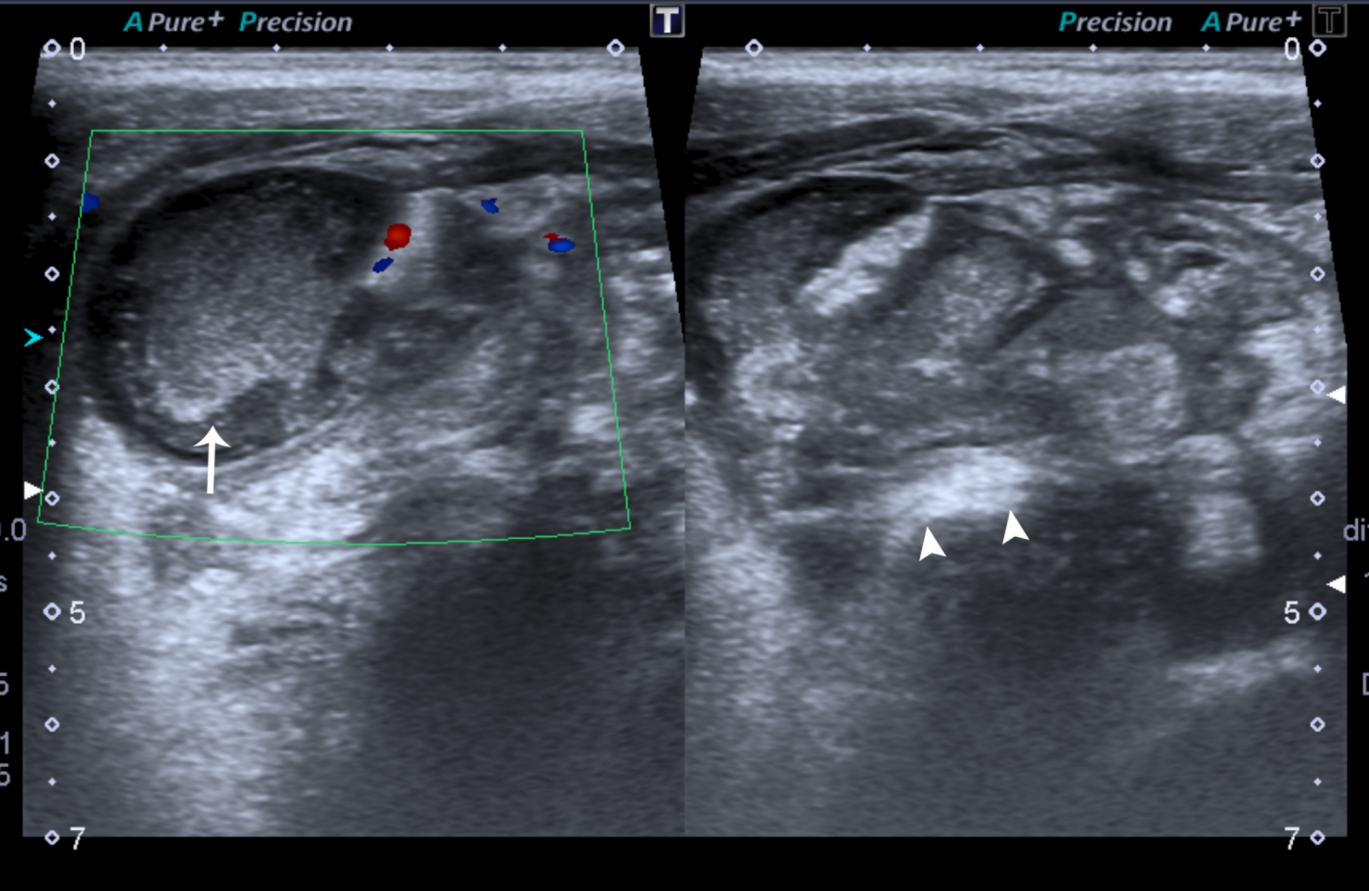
Sonographic images of a 10-year-old male A heterogenous collection with moving internal echoes (arrow) was noted in the right iliac fossa with echogenic inflammation of the surrounding fat (arrowheads). The appendix was not separately visualised. This patient had perforated appendicitis, which was confirmed on surgery.
